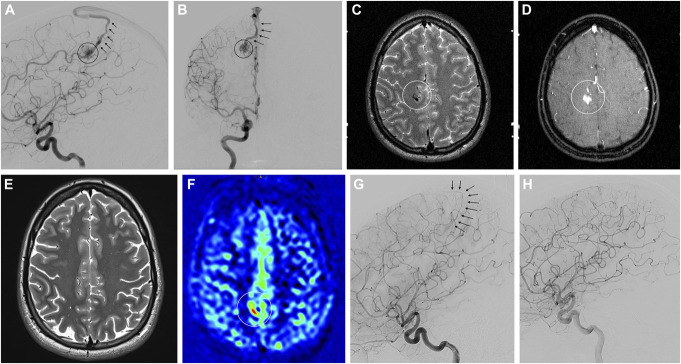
A and B, Lateral and frontal angiograms of a Spetzler-Martin 2 AVM in the right central region after right internal artery injection (circle = nidus, arrows = draining vein). The nidus was also seen on C, T2-weighted and D, time-of-flight MRI images (circle). Two years after Gamma Knife radiosurgery, the AVM was rated obliterated on E, T2w images, F, but arterial spin labeling indicated persisting AV-shunting (circle). G, An early draining vein was seen on the angiogram (arrows). However, 1 year later, H, the AVM was proven to be totally obliterated by repeat angiography without further treatment. AVM, arteriovenous malformation.
